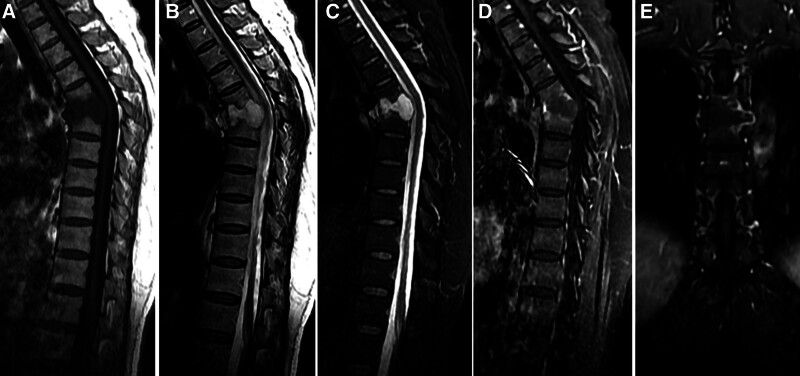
CT images of the lesion showing homogenous hypointensity on T1WI (A) and hyperintensity on T2WI (B) and STIR sequences (C). Post-contrast images show circumferential enhancement of the lesion (D, E). CT = computed tomography, STIR = short T1 inversion recovery.
